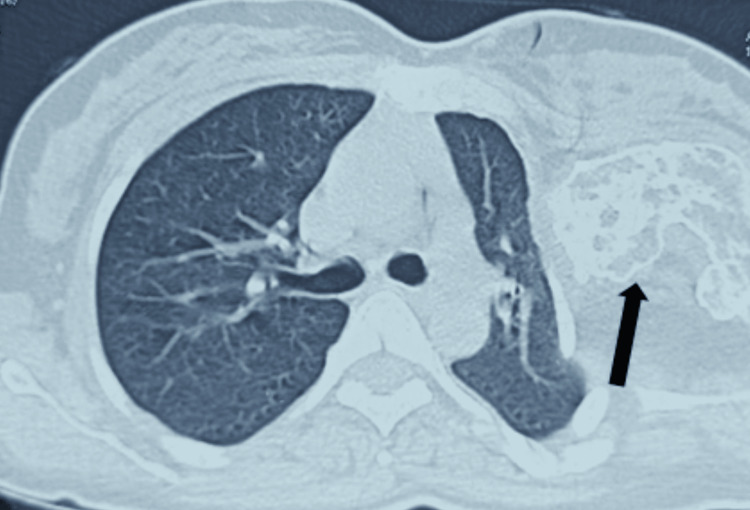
CT scan showing the compromised left lung and the tumor mass compressing the left lung CT: computed tomography
